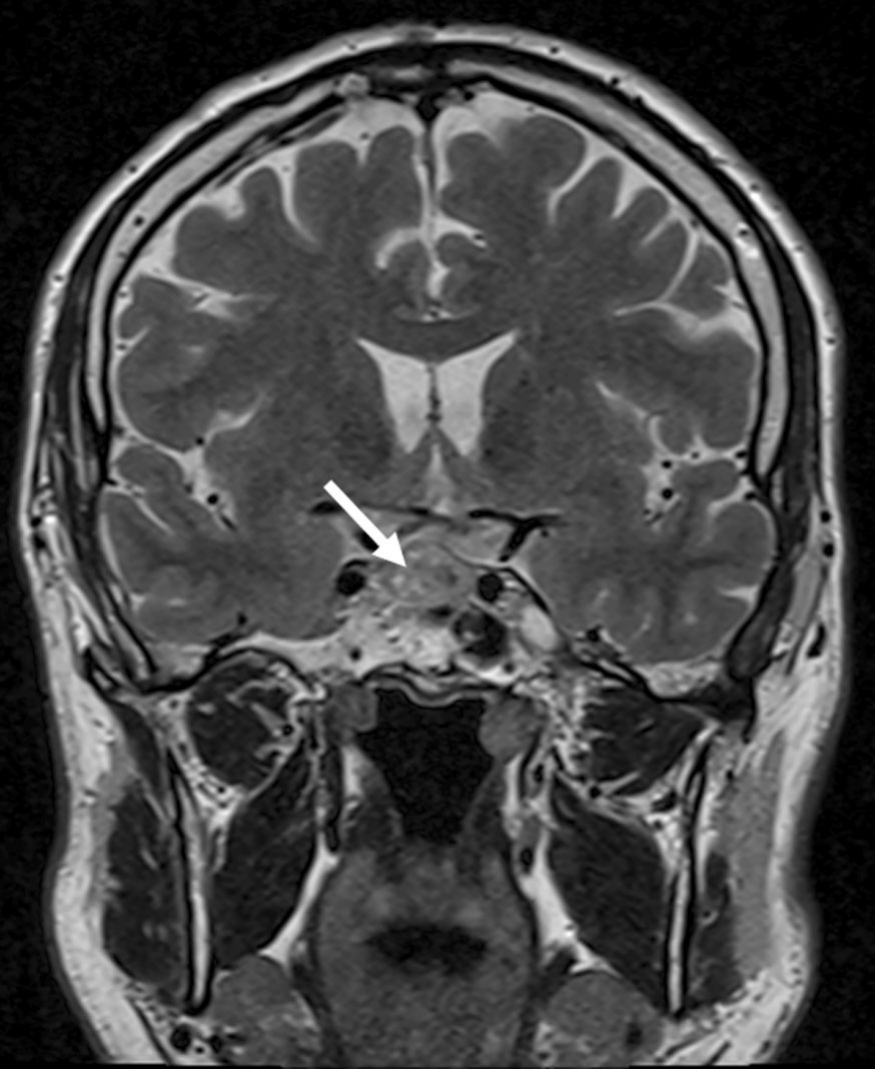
MR image showing macroadenoma (indicated by arrow)
